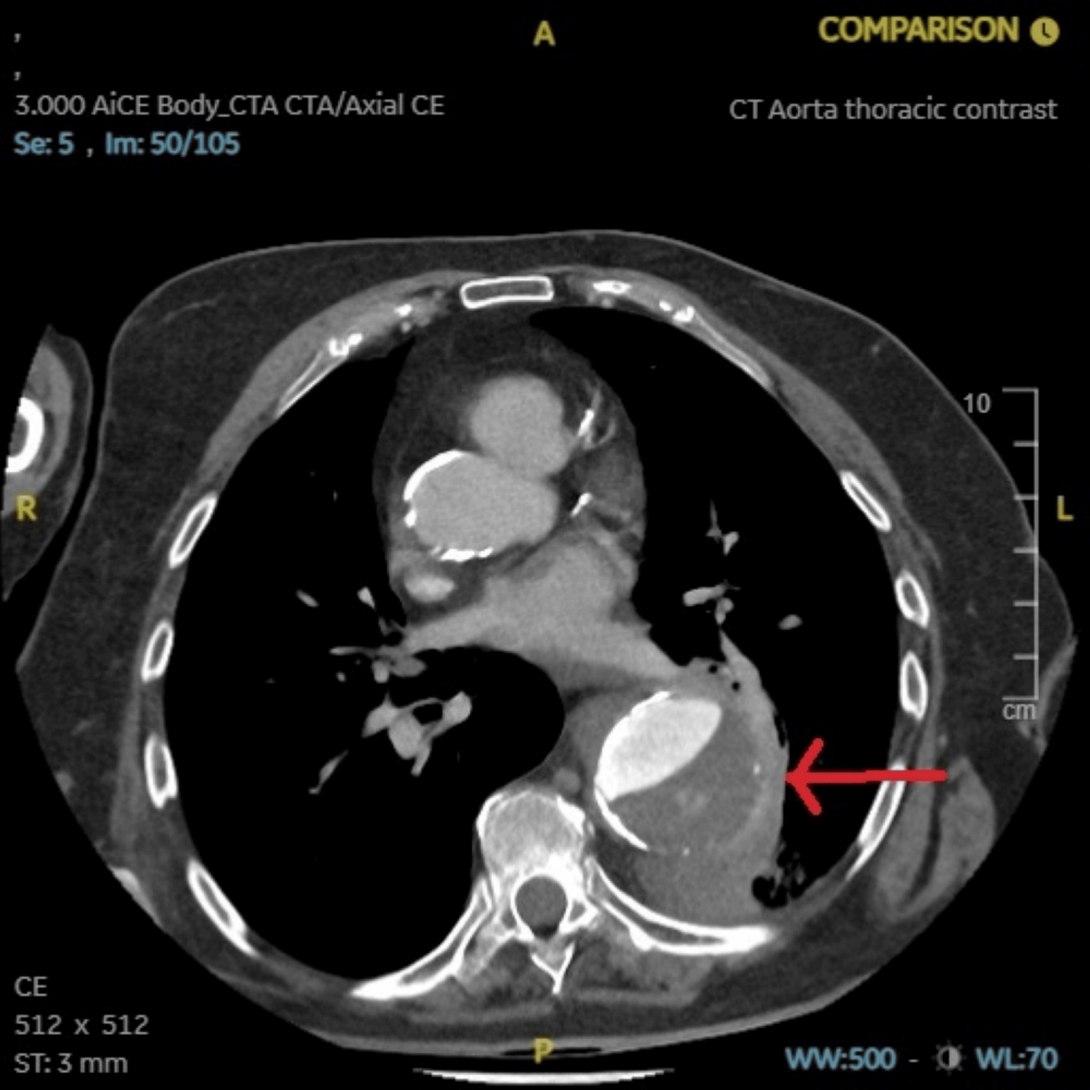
CT aorta with contrast axial view showing a 78 x 78 mm large ruptured aneurysm of the descending aorta as highlighted by the red arrow.
